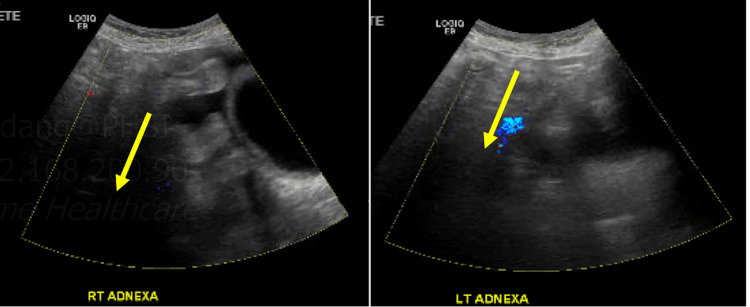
Pelvic ultrasound showing atrophic ovaries with no evidence of mass.
